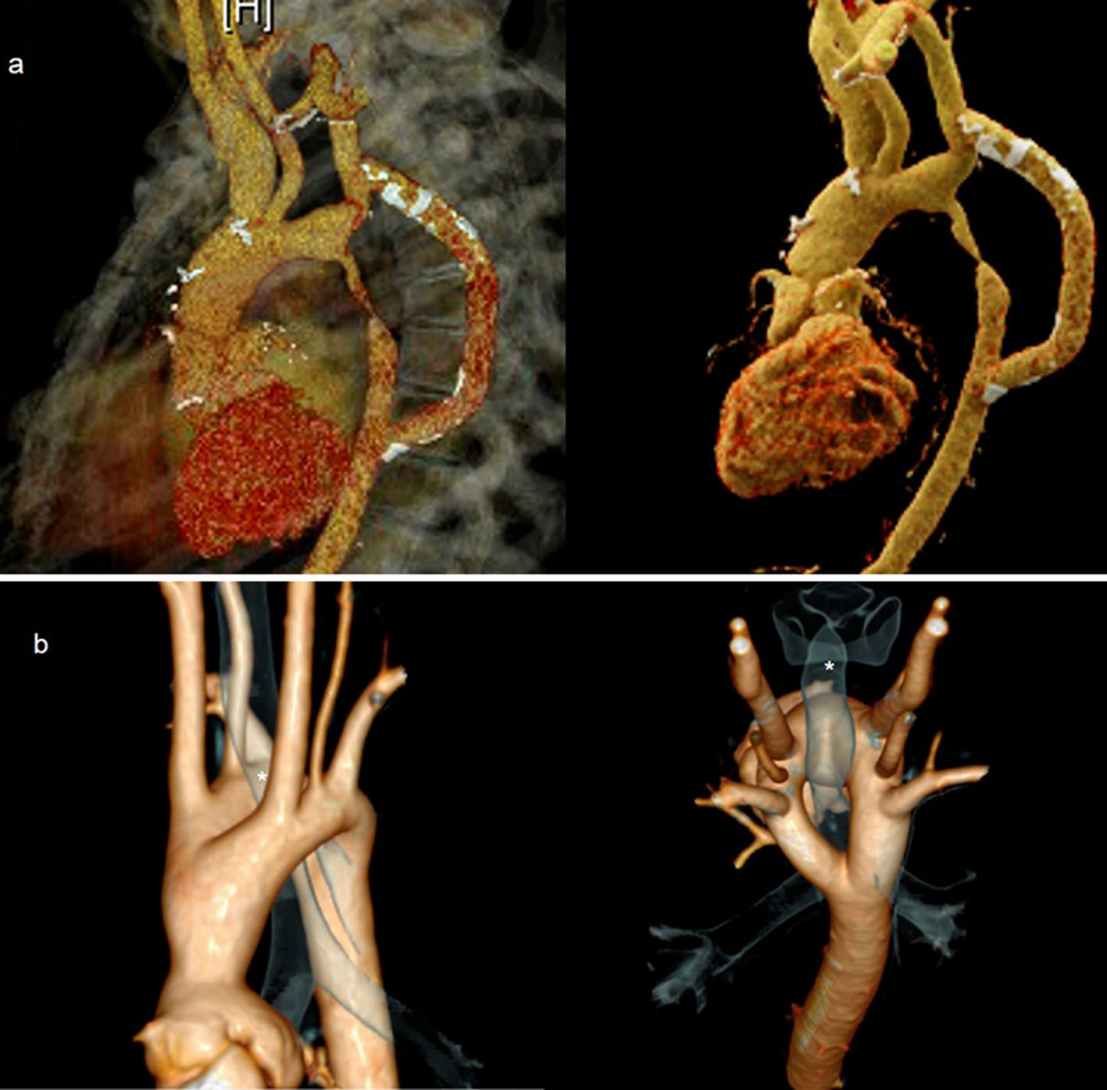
CCT 3D volume rendering images, sagittal view of a 54-year-old woman with bypass aortic coarctation palliation (a). CCT 3D volume rendering images of a double aortic arch with 3D rendering of airways structures (asterisks) showing anatomical relationships between them and the vascular ring (b). *CCT* Cardiovascular computed tomography, *3D* tridimensional
